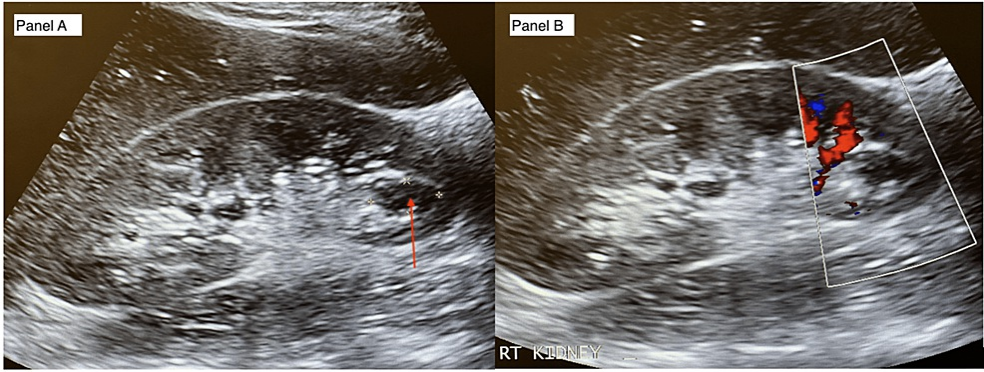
Renal cyst in the lower pole of the right kidney (red arrow) that might look like a dilated calyx (Panel A). It does not show a color Doppler effect compared to the rest of the renal pelvis (Panel B).
